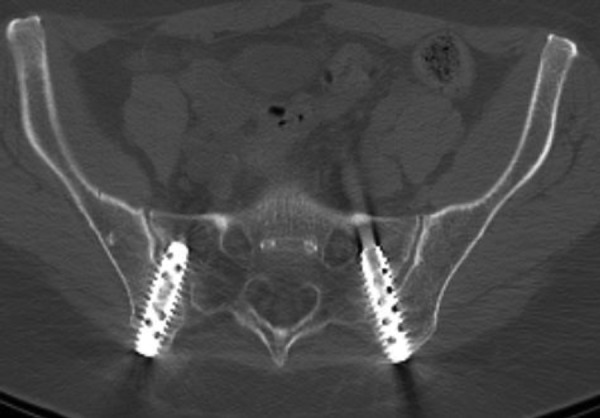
Axial CT showing trajectory of procedure
